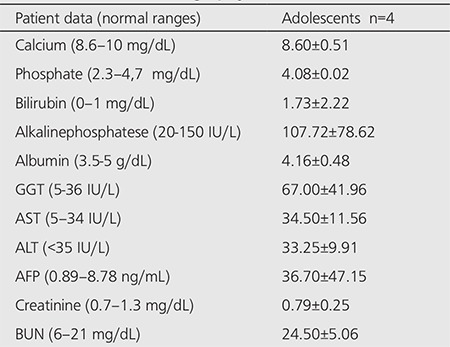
Biochemical finding in adolescent patients atthe time of bone scintigraphy
